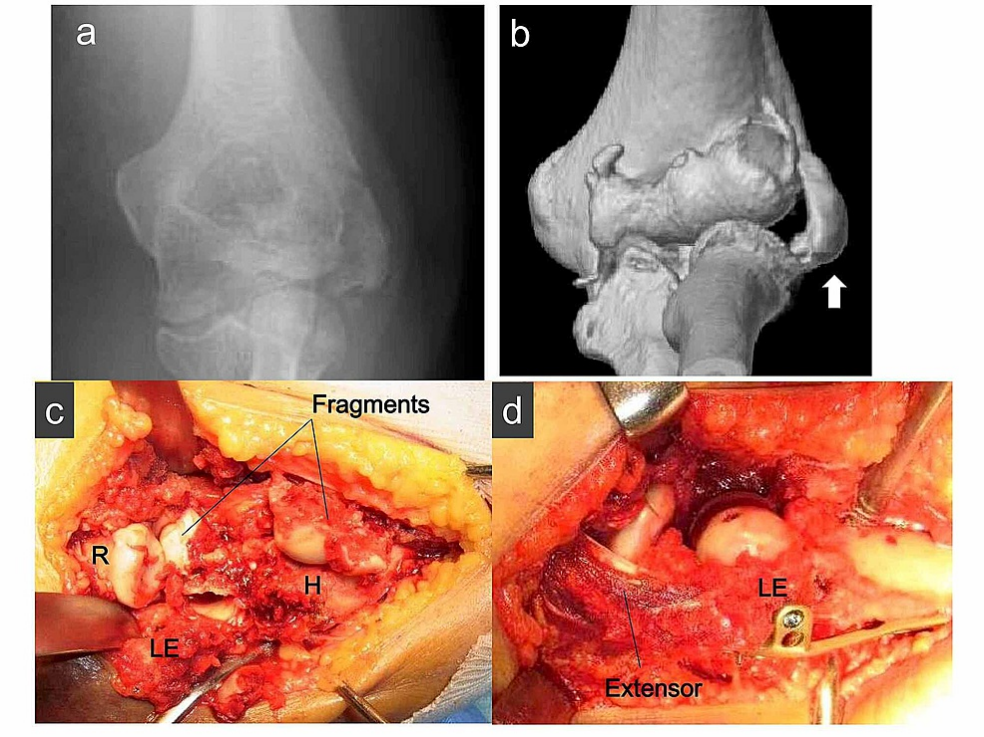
(a) Preoperative anteroposterior X-ray and (b) CT scan showing a lateral epicondyle fracture (white arrow) by Dubberley type 3B. (c) The lateral epicondyle fragment is retracted distally with the extensor origin. The elbow joint is exposed with the extensile Kocher approach. (d) Capitellum and trochlea fragments are fixed with anteroposterior headless screws after the lateral epicondyle fragment is fixed with a locking plate. R: radial head, H: distal humerus, LE: lateral epicondyle
